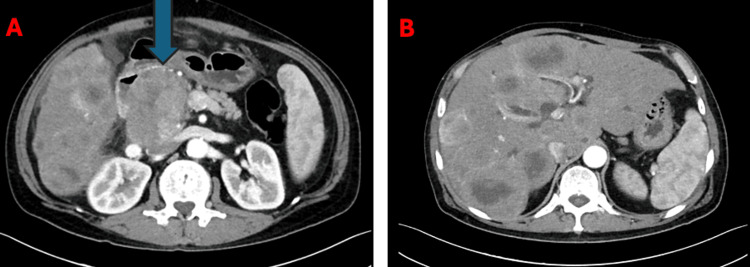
(A) CT of the abdomen showing a large mass in the pancreaticoduodenal groove (blue arrow). (B) CT of the abdomen showing multiple lesions in the liver, suggestive of liver metastases.
